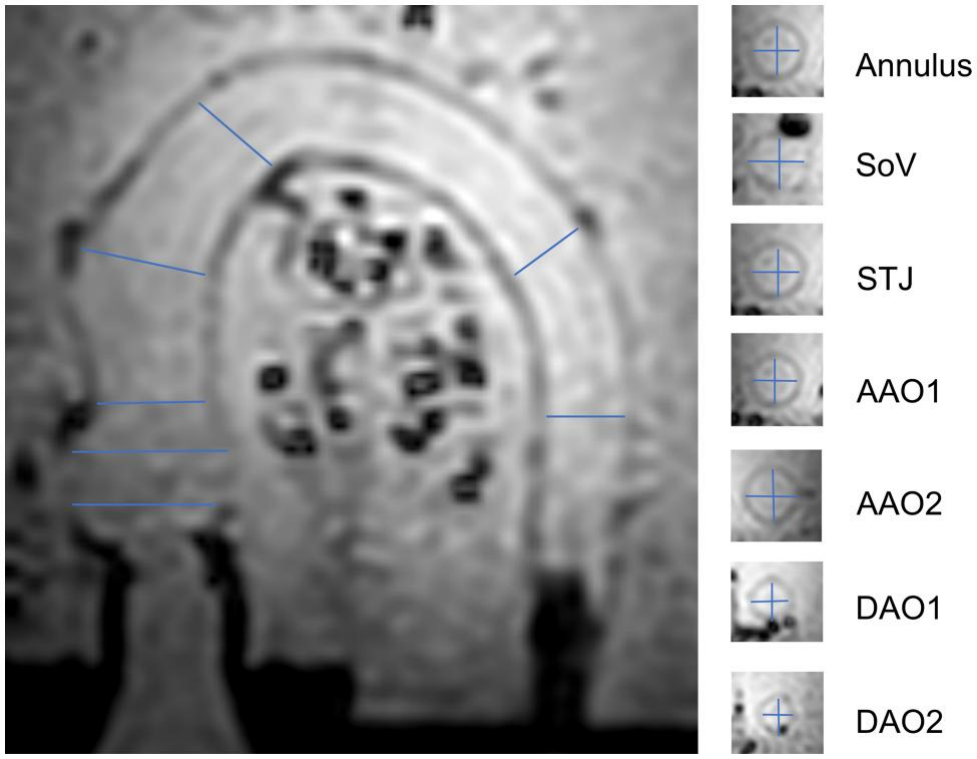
4D flow MRI-derived magnitude image, an example of measurement at the annulus, the sinus of Valsalva (SoV), the sinutubular junction (STJ), the ascending aorta (AAO1, AAO2) and the descending aorta (DAO1, DAO2)
